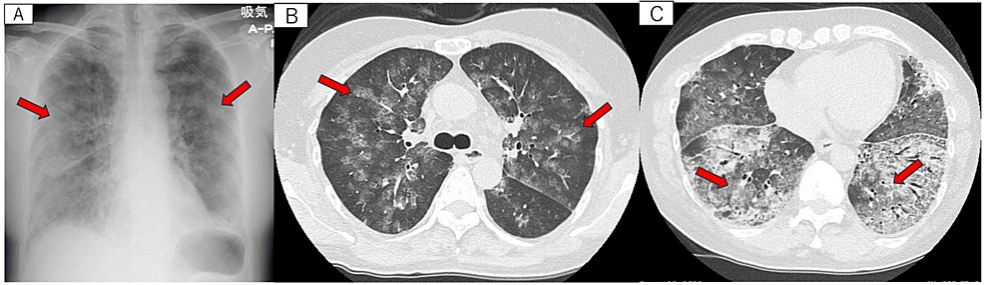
Chest X-ray and computed tomography after the bronchoscopy. Chest X-ray shows bilateral diffuse infiltrative shadows (A). The computed tomography reveals new bilateral infiltrates, primarily central in location. It also shows thickening of the interlobular septa and patchy shadows (B,C). Each red arrow indicates new ground-glass opacity.
